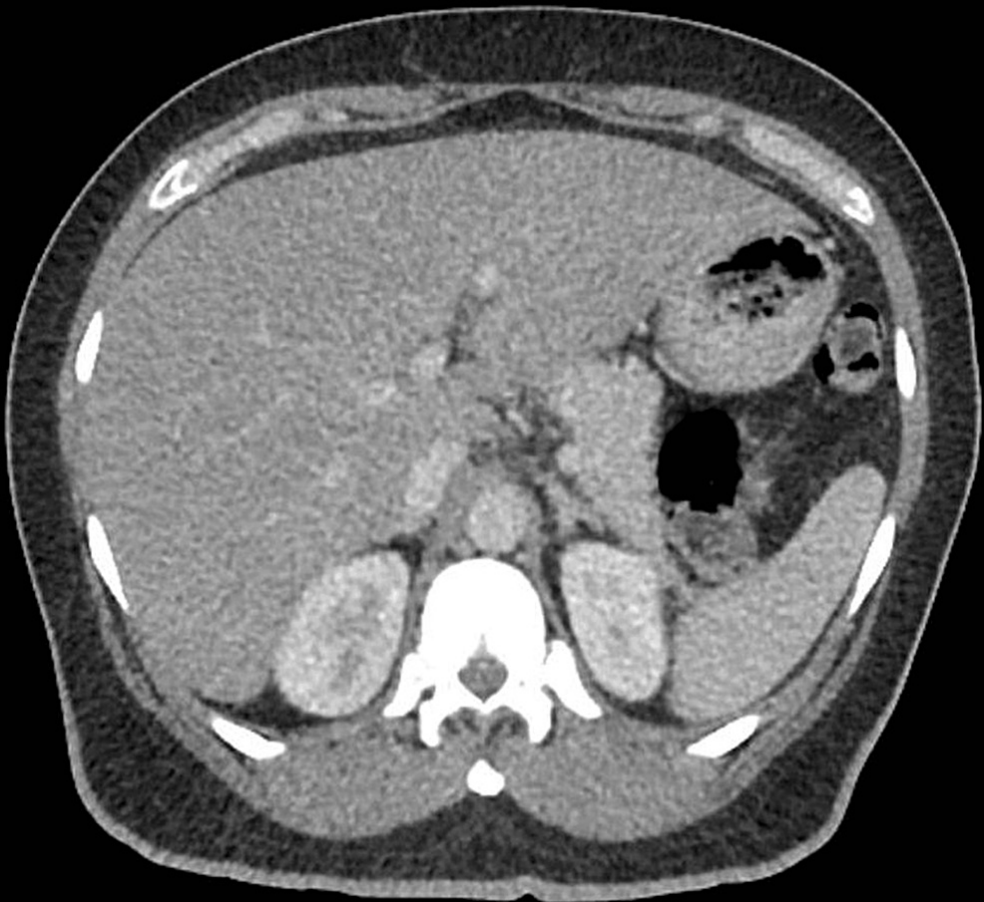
Abdominal computer tomography (CT) findings: hepatomegaly with diffuse hepatic steatosis.
